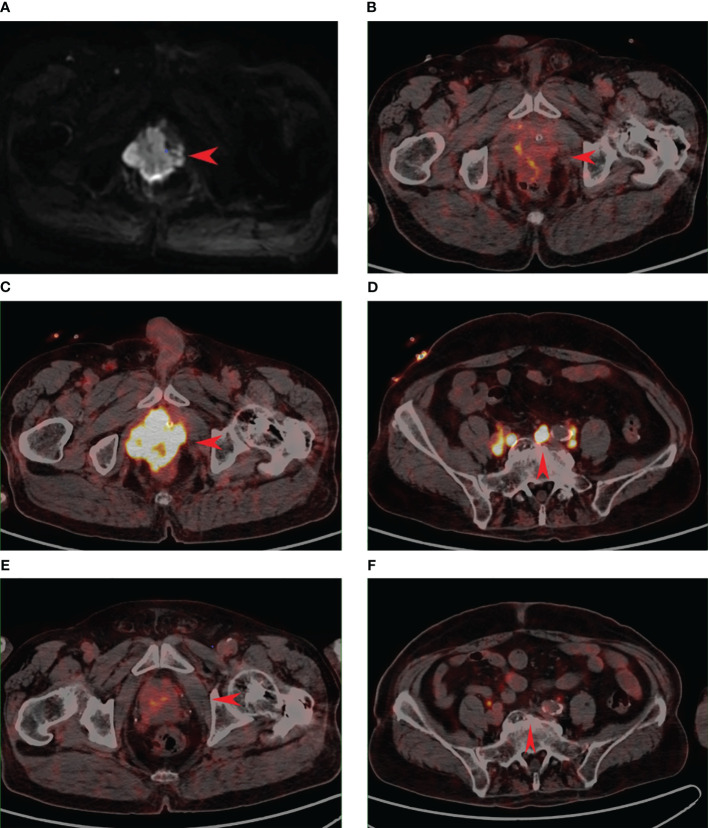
A.2022.5 Prostate MR scan: review after prostate cancer treatment to consider disease progression B.2022.5 PSMA PET suggests an irregularly enlarged prostate with heterogeneous density, uneven PSMA radioactivity distribution only slightly increased, SUVmax=4.8. (B) is inconsistent with the imaging performance of the lesion in (A). C.2022.5 18F-OC PET suggests foci of high octreotide expression in the prostate, SUVmax=12.3. (C) is consistent with the imaging presentation of the lesion in (A). D.2022.5 Pelvic lymph node under 18F-OC PET, increased distribution of radioactivity is seen, SUVmax=6.2. (E) 18F-OC PET image 3 months after 177Lu-DOTA-TATE treatment, the volume of the lesion is significantly reduced compared with that before the treatment, and the level of octreotide expression is significantly reduced compared with the previous one, which is considered to be the residual tumor activity. SUVmax=5.5. (F) 18F-OC PET image 3 months after 177Lu-DOTA-TATE treatment, pelvic lymph nodes compared with (D), the expression level of octreotide was significantly reduced, which was consistent with post-treatment changes.
